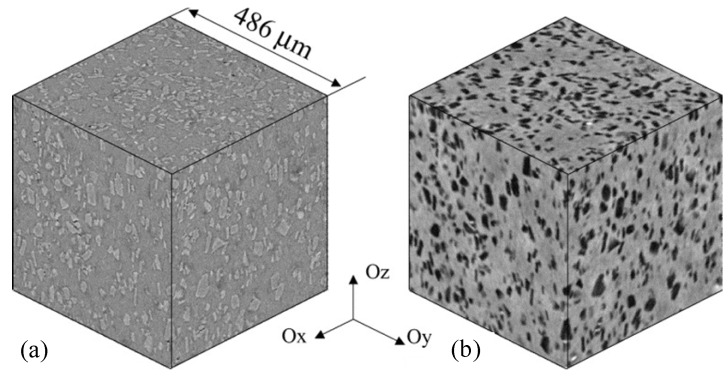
Reconstructed microstructure of 20 vol% Al_2_O_3_ reinforced Al 6061 MMC with two types of X-ray microcomputed tomography. (a) Phase-contrast tompography; and (b) holotomography [66].
